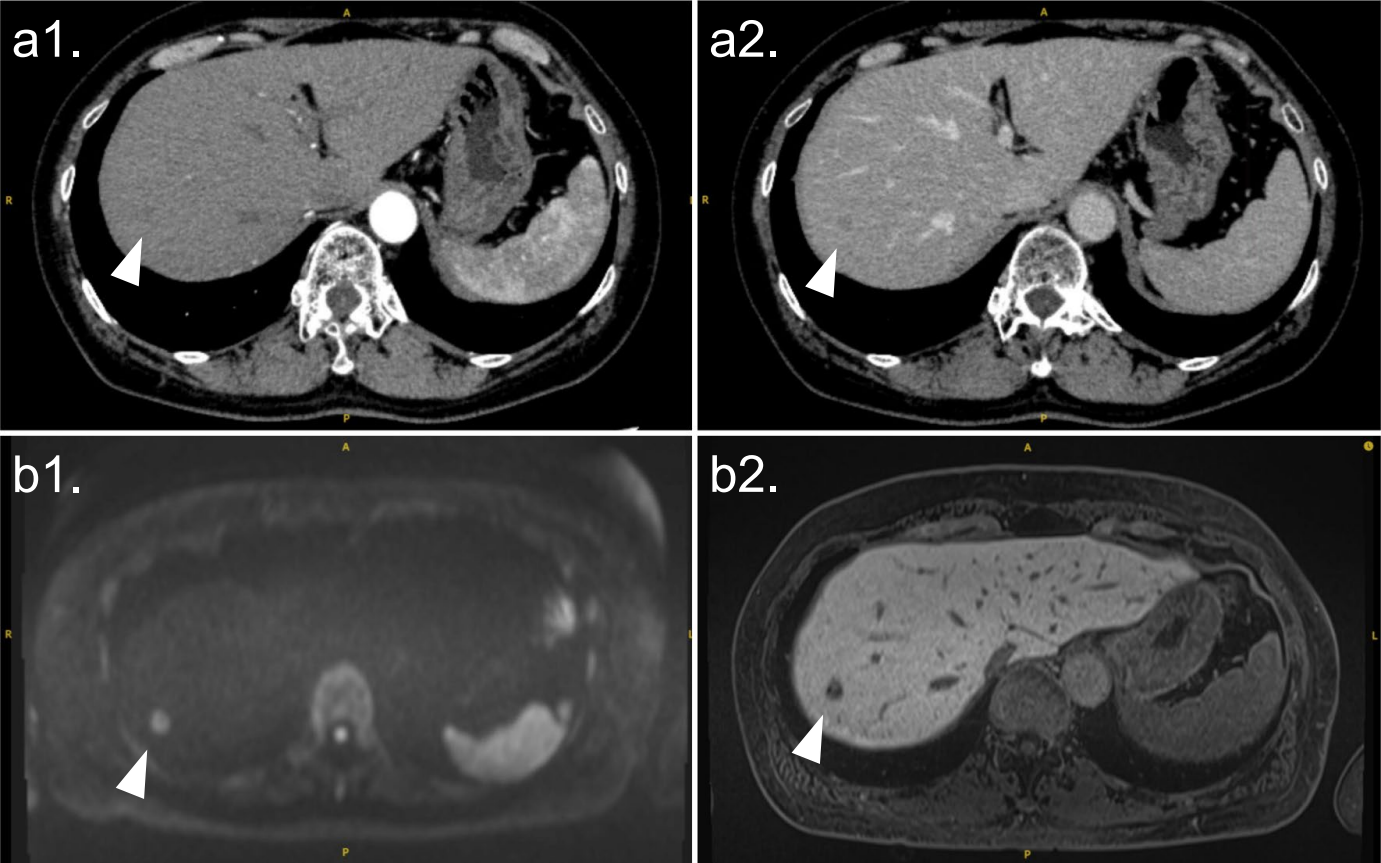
Preoperative imaging examinations. MDCT shows a low-density nodule, about 1 cm in diameter during the arterial phase (a1) and ring enhancement during the delayed phase (a2) in liver segment 8. EOB-MRI shows restricted diffusion (b1) and a low-signal intensity mass during the hepatobiliary phase (b2) in liver segment 8. *MDCT* multidetector computed tomography, *EOB-MRI* Gd-EOB-DTPA-enhanced magnetic resonance imaging.
